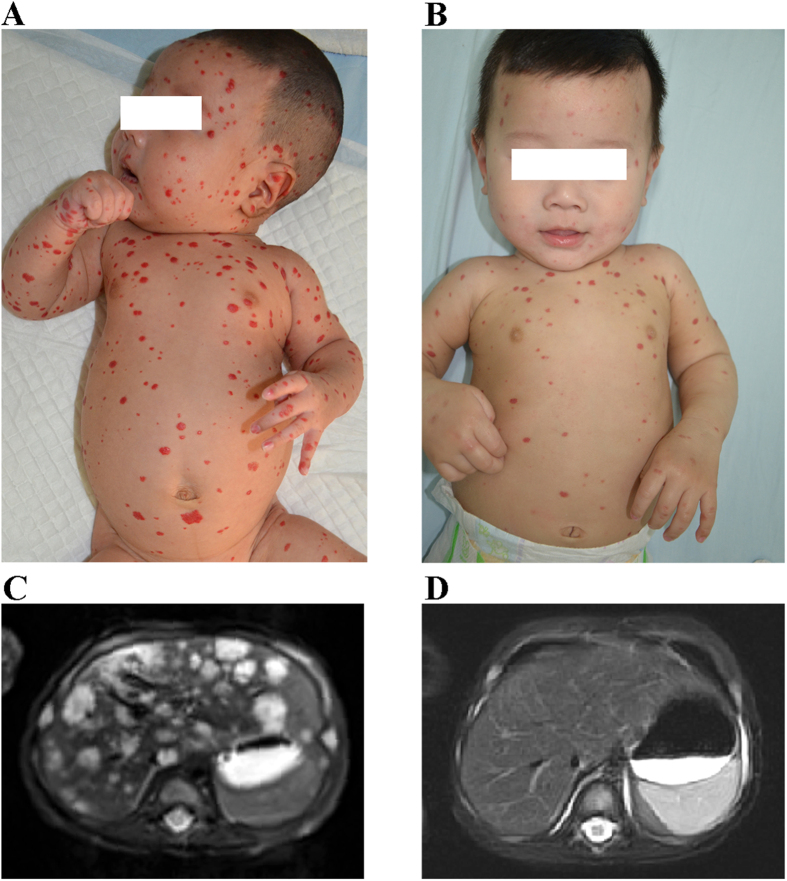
Propranolol treatment for multifocal IH in a 1.5-month-old boy (Patient #11 in Table 2). Clinical photographs of cutaneous IHs, 1 day before propranolol treatment (A) and 6 months after the start of treatment (B). T2-weighted axial MRI showed MHH, 1 day before propranolol treatment (C) and 6 months after the start of treatment (D).
